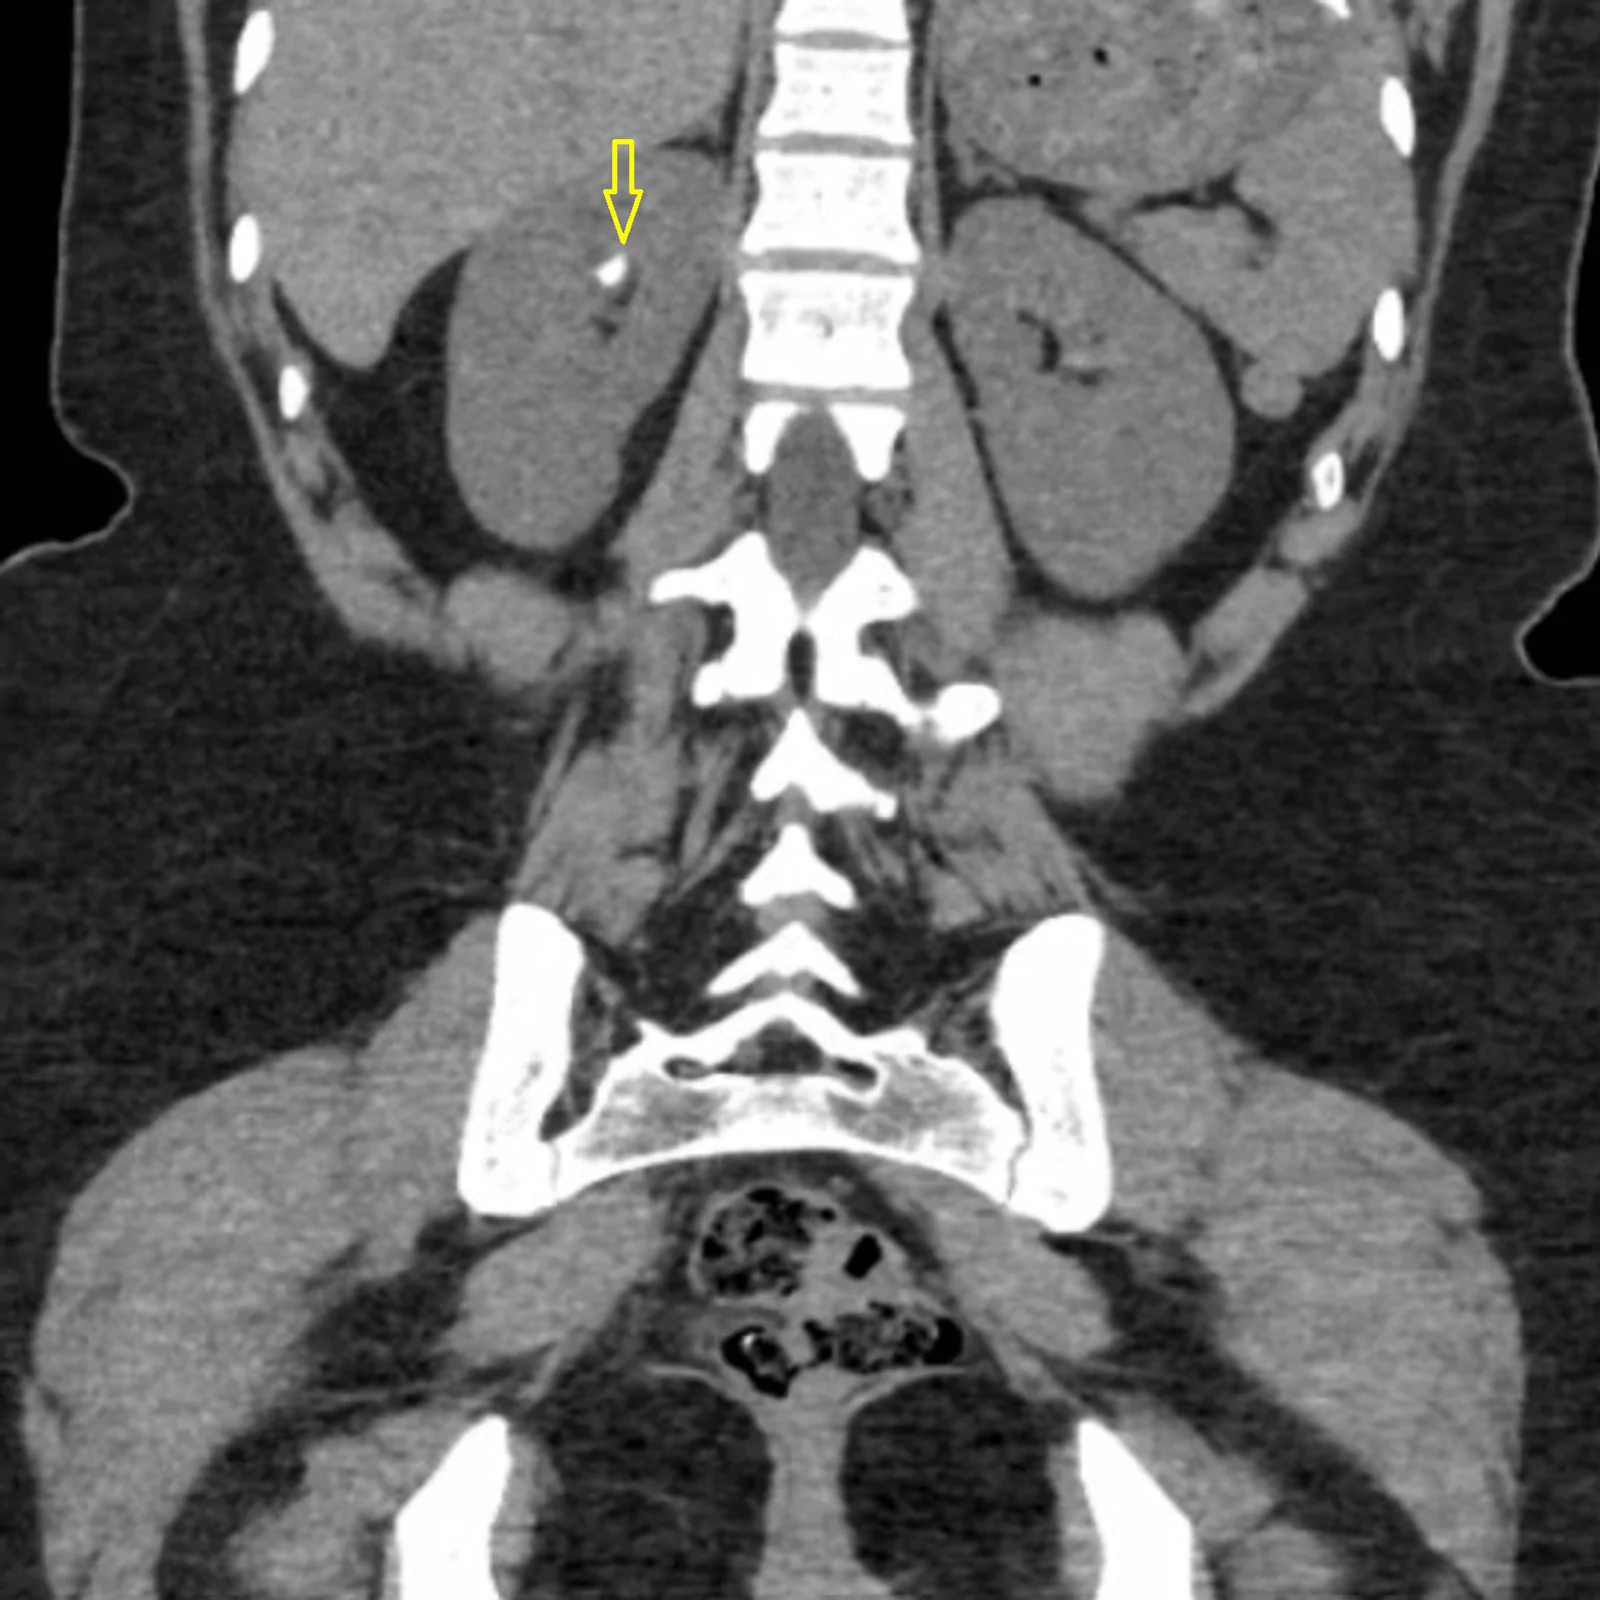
CT scan of the abdomen/pelvis CT scan of the abdomen/pelvis, renal stone protocol (without contrast), coronal view, demonstrating nephrolithiasis of the right kidney (yellow arrow).
